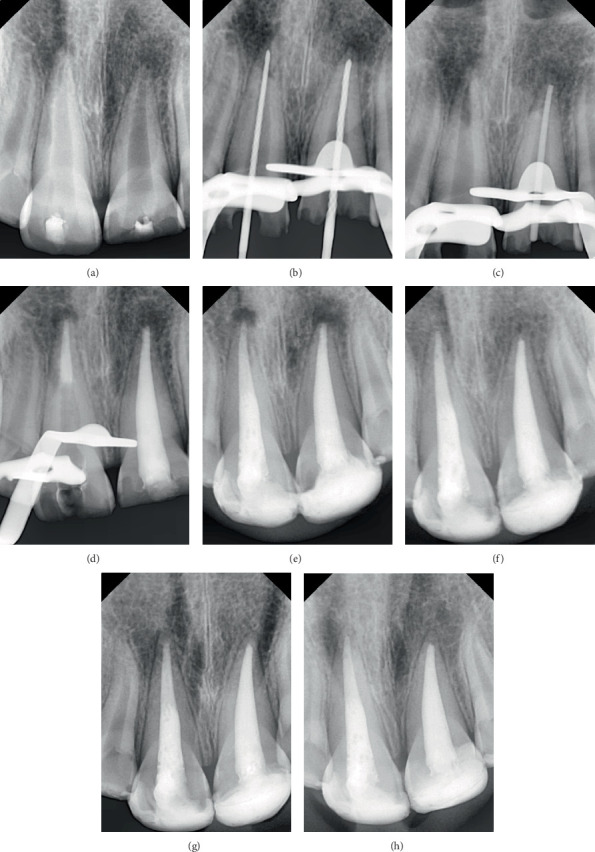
Case 4. (a) Preoperative periapical radiograph. (b) Working length radiograph. (c) Cone fit radiograph (Tooth #21). (d) Intermediate radiograph showing apical plug formation in Tooth #11 and obturation of Tooth #21. (e) Postoperative radiograph. (f) A 6-month recall radiograph. (g) A 12-month recall radiograph. (h) A 24-month recall radiograph.
